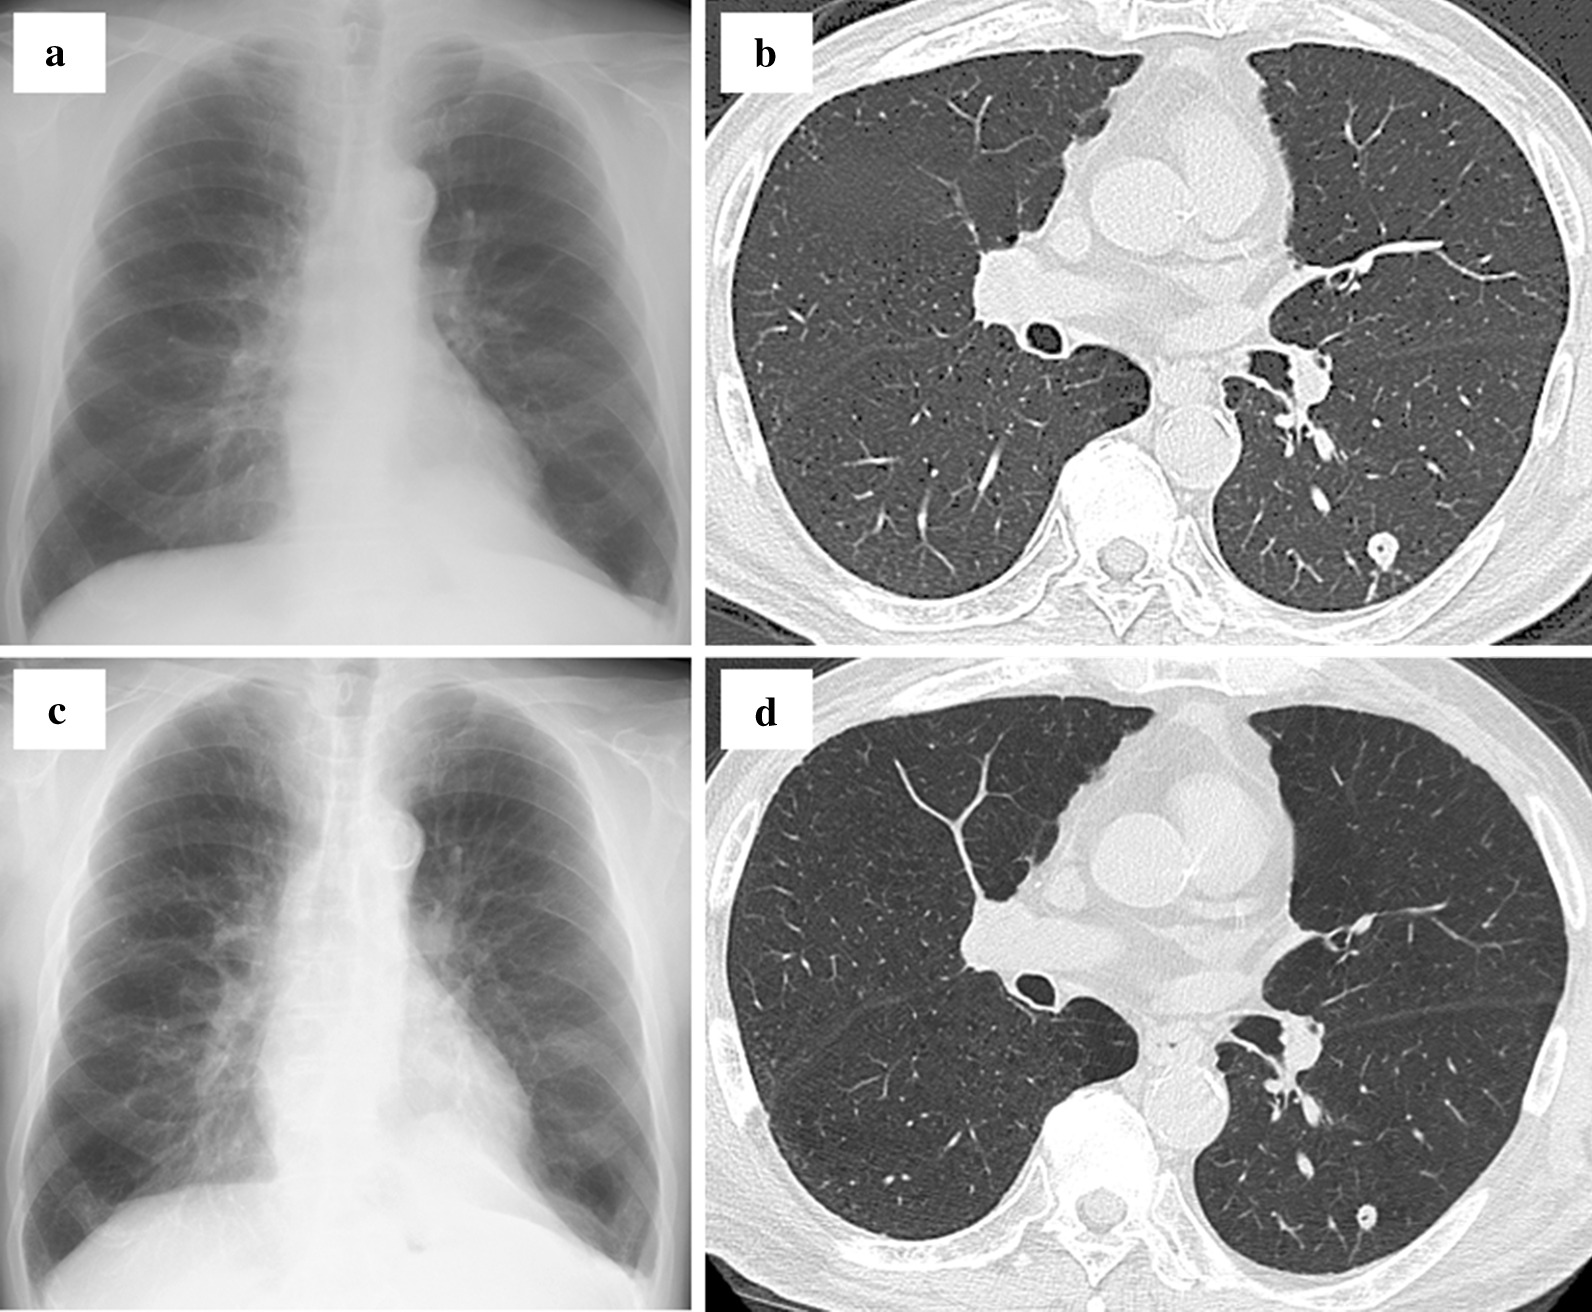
Chest X-ray and computed tomography (CT). a Chest X-ray on admission showing a solitary nodule in the left middle lung field. b Chest CT on admission showing a 12-mm nodule with a cavitary lesion and a centrilobular branching structure (tree-in-bud appearance) in the lower lobe (S^6^) of the left lung. c Chest X-ray 9 months after treatment initiation showing decreased size of the solitary nodule in the left middle lung field. d Chest CT 9 months after treatment initiation showing a XX-mm nodule diminishing the tree-in-bud appearance
